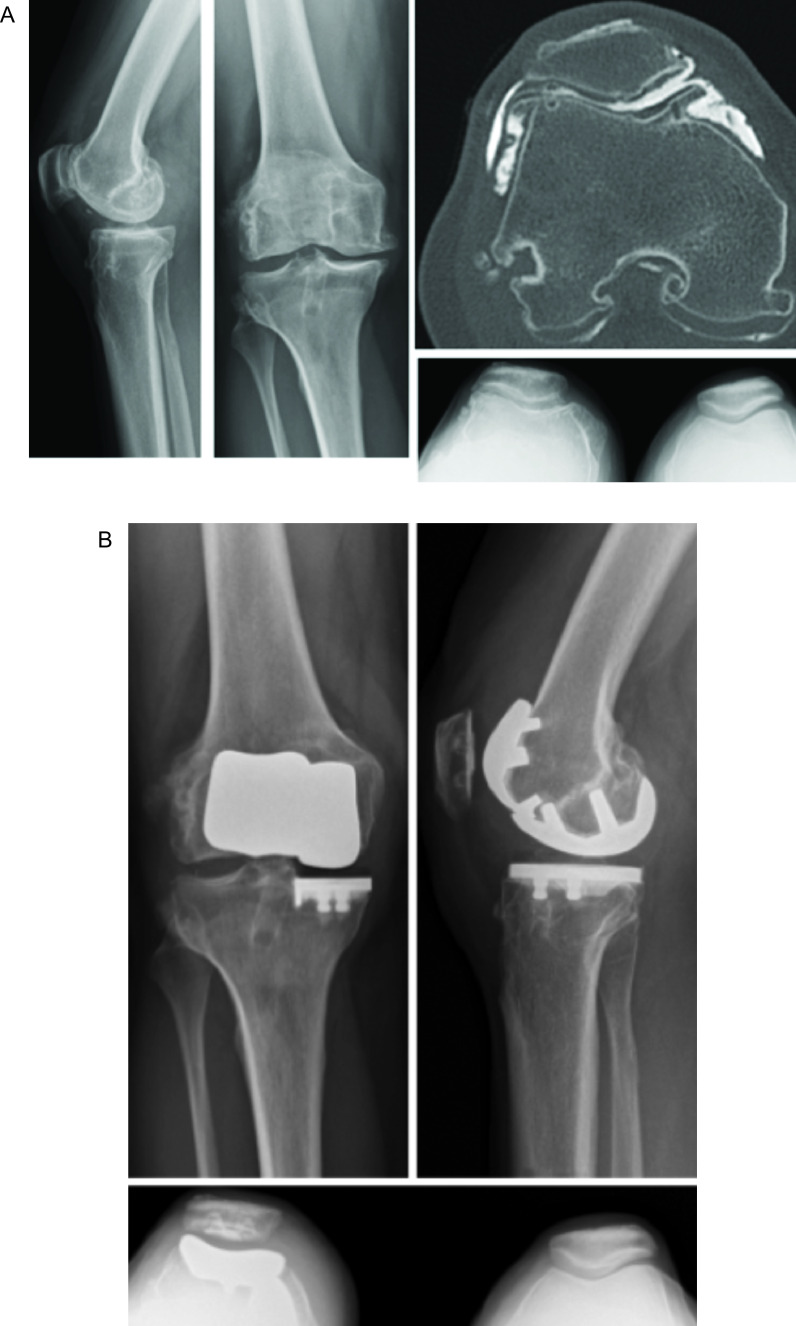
Woman of 48 years old with a prior history of ACL reconstruction 30 years ago. She is an active patient and is now limited by medial tibiofemoral, and patellofemoral osteoarthritis confirmed on X-rays and Arthro-CT scan (A). Physical examination found no anterior laxity and no pain in the lateral compartment. Patellofemoral and medial unicompartmental knee arthroplasty were performed simultaneously with robotic assistance (B). The patient was very satisfied at 2 years follow-up with no residual pain and returned to a normal life.
